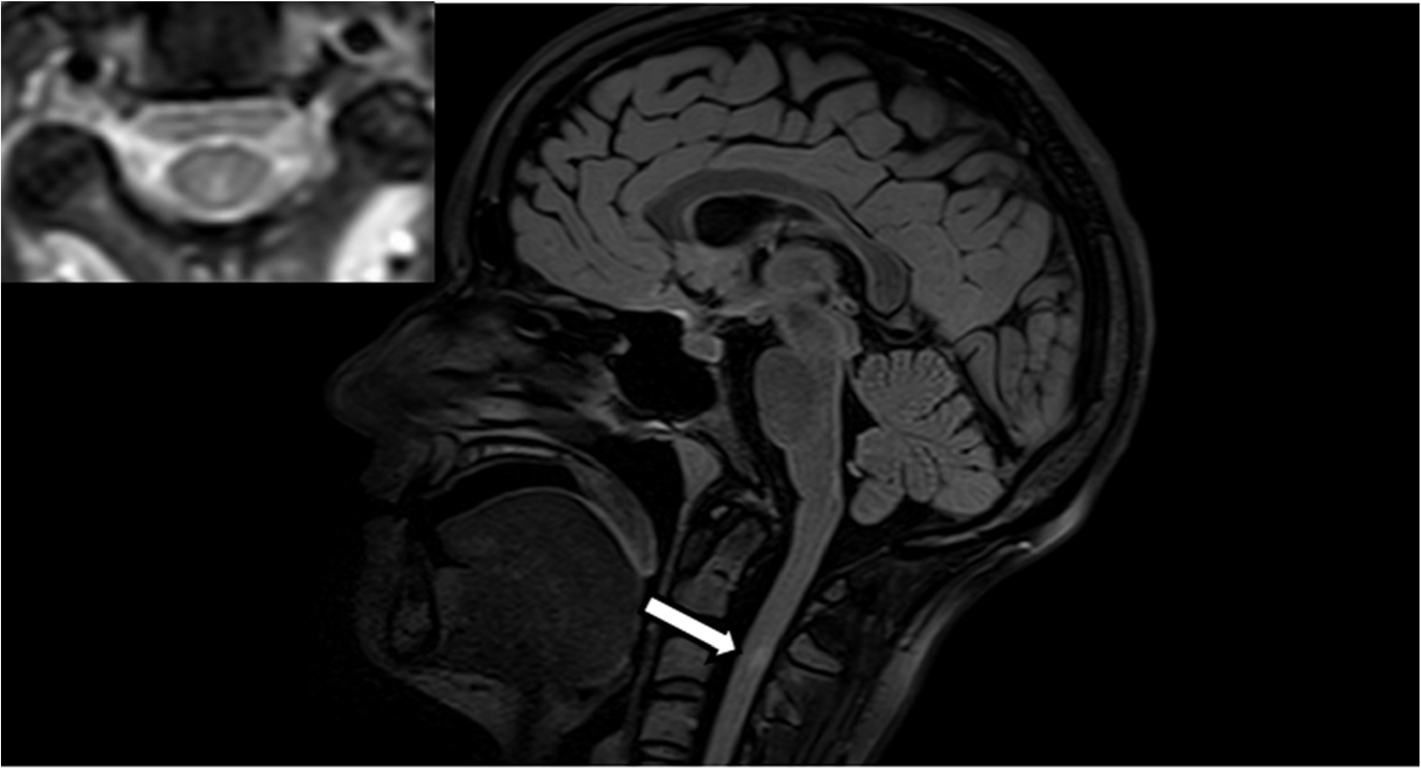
MRI image (FLAIR sagittal and T2 transversal plane), showing the lesion in the dorsal column of the cervical spinal cord C2/C3
